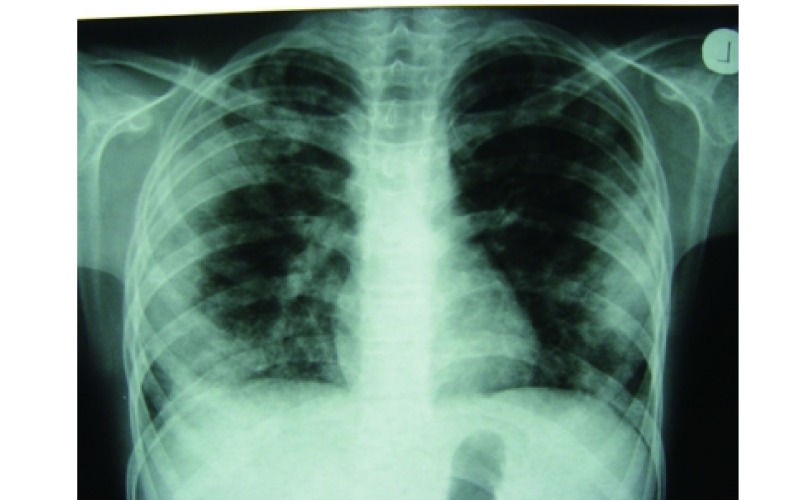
Chest X-ray and CT-scan of thor
